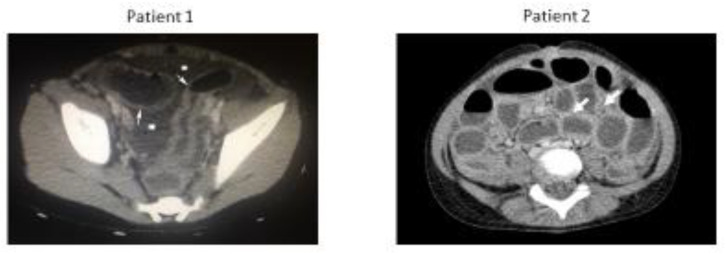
Contrast-enhanced transverse computed tomography imaging view of the abdomen of Patients 1 and 2. Free intraperitoneal fluid (asterisks) and intestinal wall thickening (arrows) can be seen.
